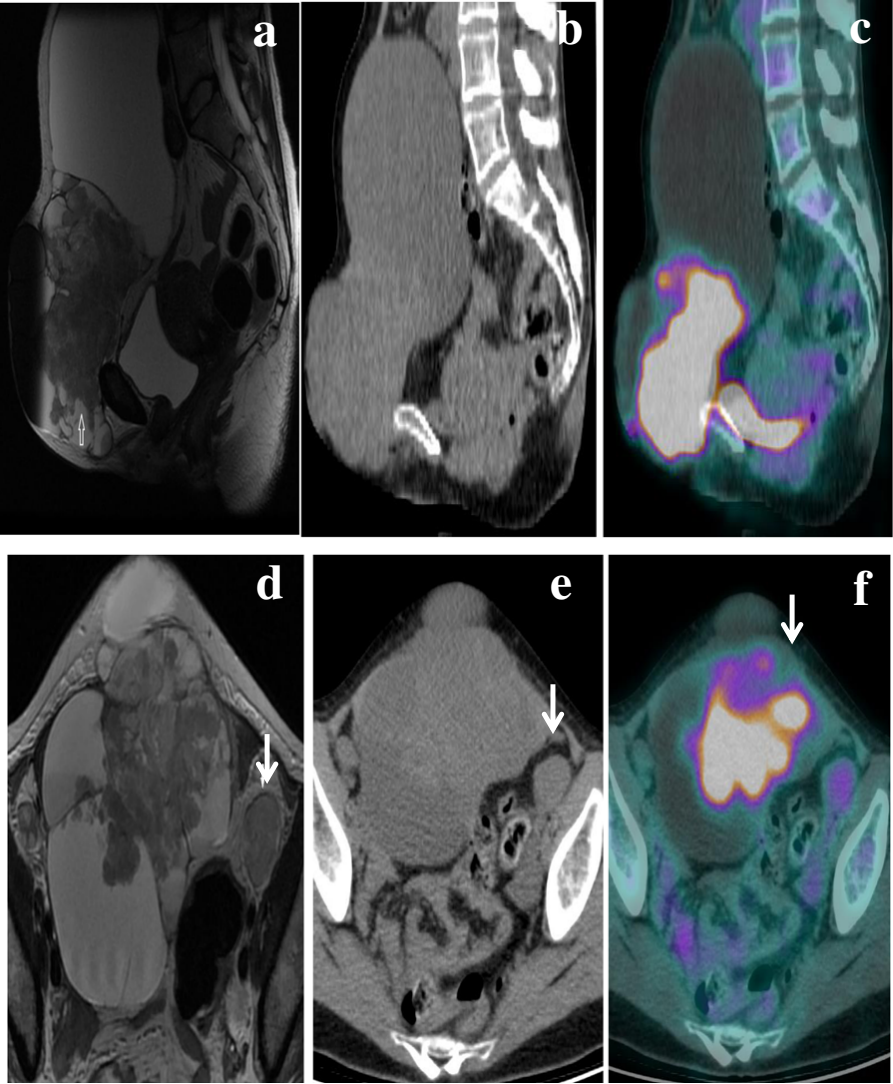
Pre-surgical T2-weighted FSE sagittal MRI (a) documenting a supra-vesical pelvic mass extending into the anterior pelvic wall and showing non-homogeneous signal intensity and solid components. Sagittal CT (b) and fused ^18^F-FDG PET/CT (c) images showed non-homogeneous uptake in the 22 cm abdominal mass: in particular, intense abnormal ^18^F-FDG uptake was present in the caudal solid component of the mass, whereas an absent area of tracer uptake was evident in its cranial fluid component. Corresponding T2-weighted FSE axial MRI (d) confirmed the presence of a solid and partially fluid pelvic mass, and enlarged loco-regional lymph nodes (*arrow*). Axial CT (e) and fused ^18^F-FDG PET/CT images (f) showed intense tracer uptake in the solid component of the mass, extended up to the skin surface of pelvis, and enlarged left external iliac lymph-node, characterized by increased tracer uptake (*arrows*)
